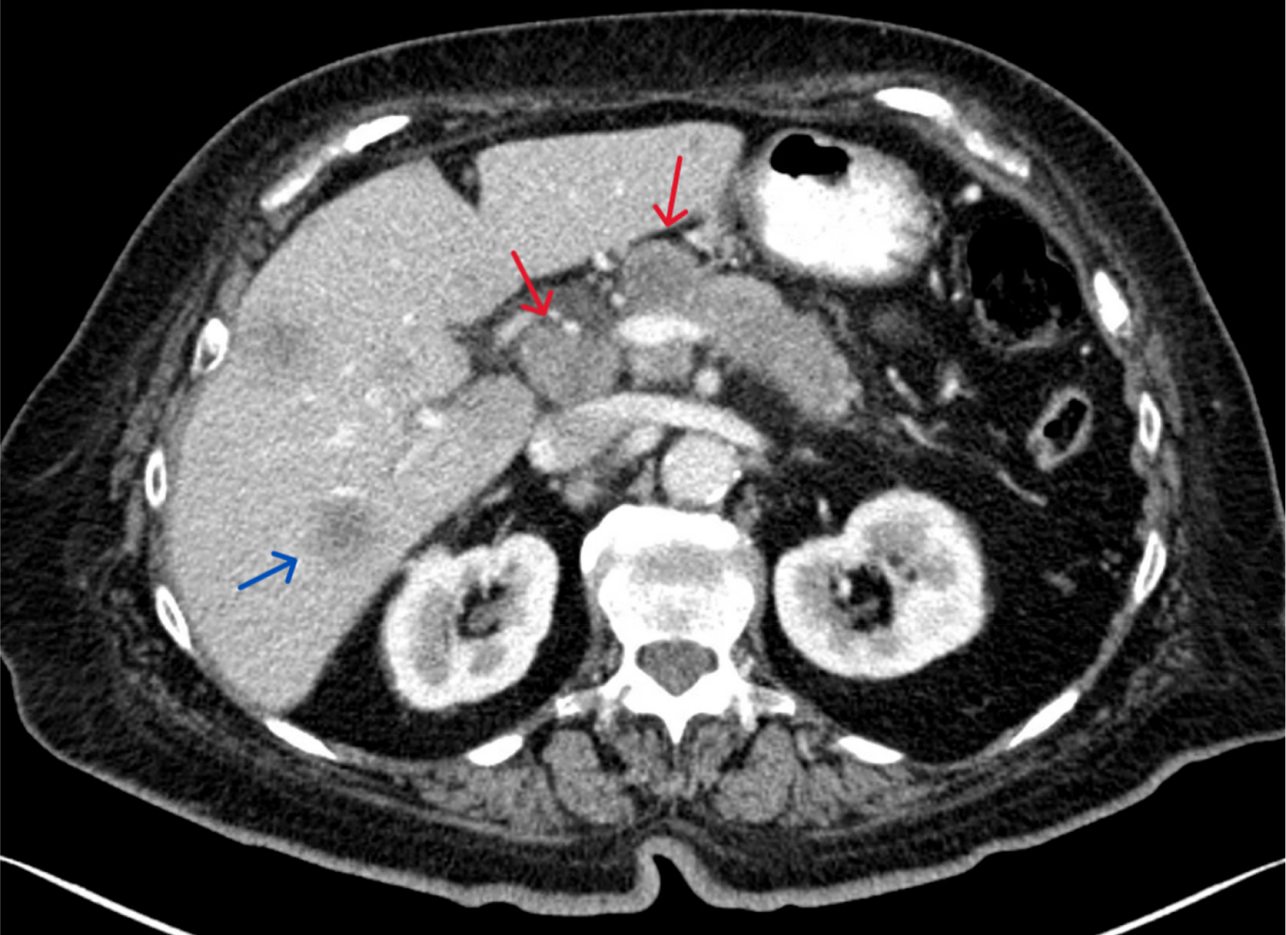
Axial post‐contrast CT image demonstrates the heterogeneously enhancing mass in the pancreatic head and proximal body (red arrows), compatible with the known primary neuroendocrine tumor. Incidentally, hepatic metastases are also partially seen (blue arrow).
